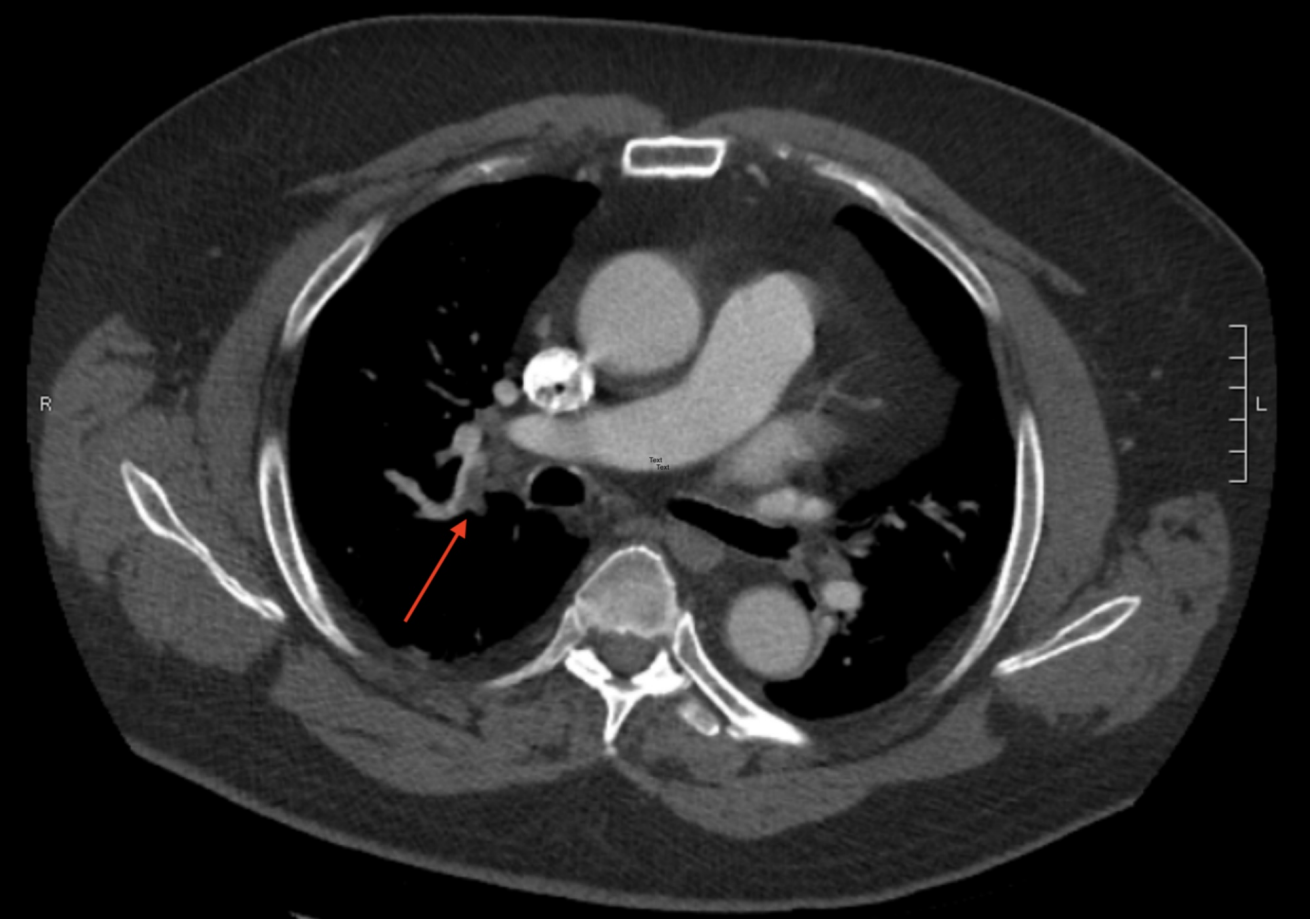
PE protocol CT scan showing a PE in the right pulmonary artery. PE: Pulmonary Embolism
